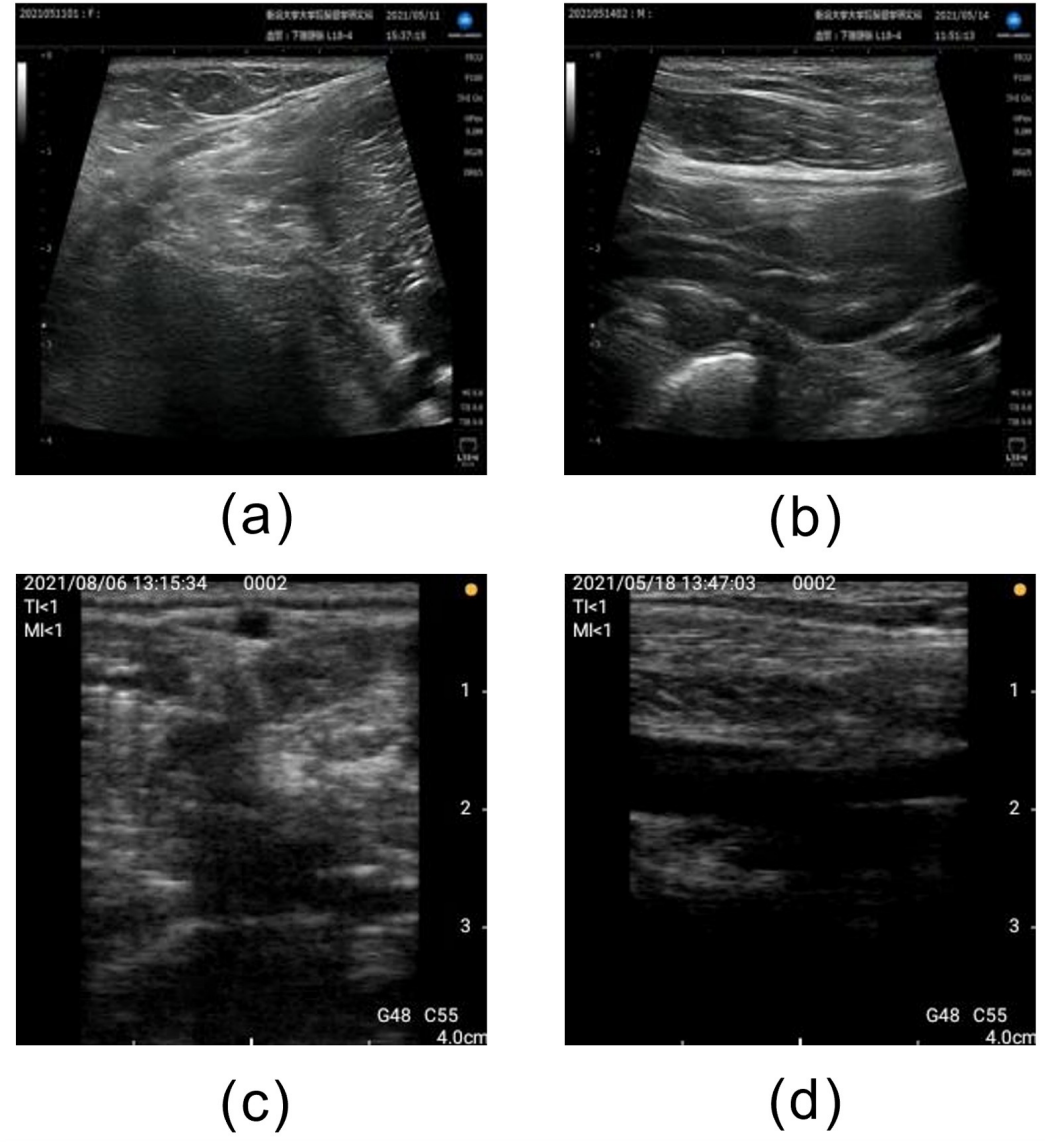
Examples of correctly classified Moderately Satisfactory images. (a) Short axis acquired by stationary ultrasonography equipment, (b) Long axis acquired by stationary ultrasonography equipment, (c) Short axis acquired by portable ultrasonography equipment, and (d) Long axis acquired by portable ultrasonography equipment.
